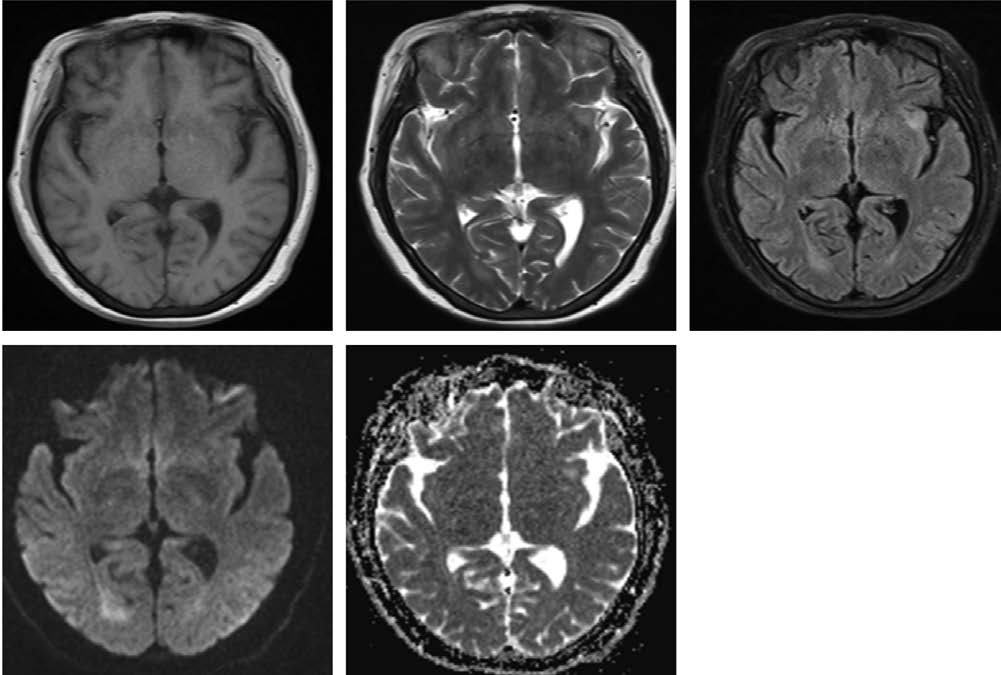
The right occipital lobe and left insular lobe showed patchy slightly longer T1 and slightly longer T2 signals, slightly high signal on FLAIR sequence, limited diffusion on DWI, and high signal on ADC. ADC = apparent diffusion coefficient, DWI = diffusion-weighted imaging, FLAIR = fluid attenuated inversion recovery, T1 = T1-weighted imaging, T2 = T2-weighted imaging.
